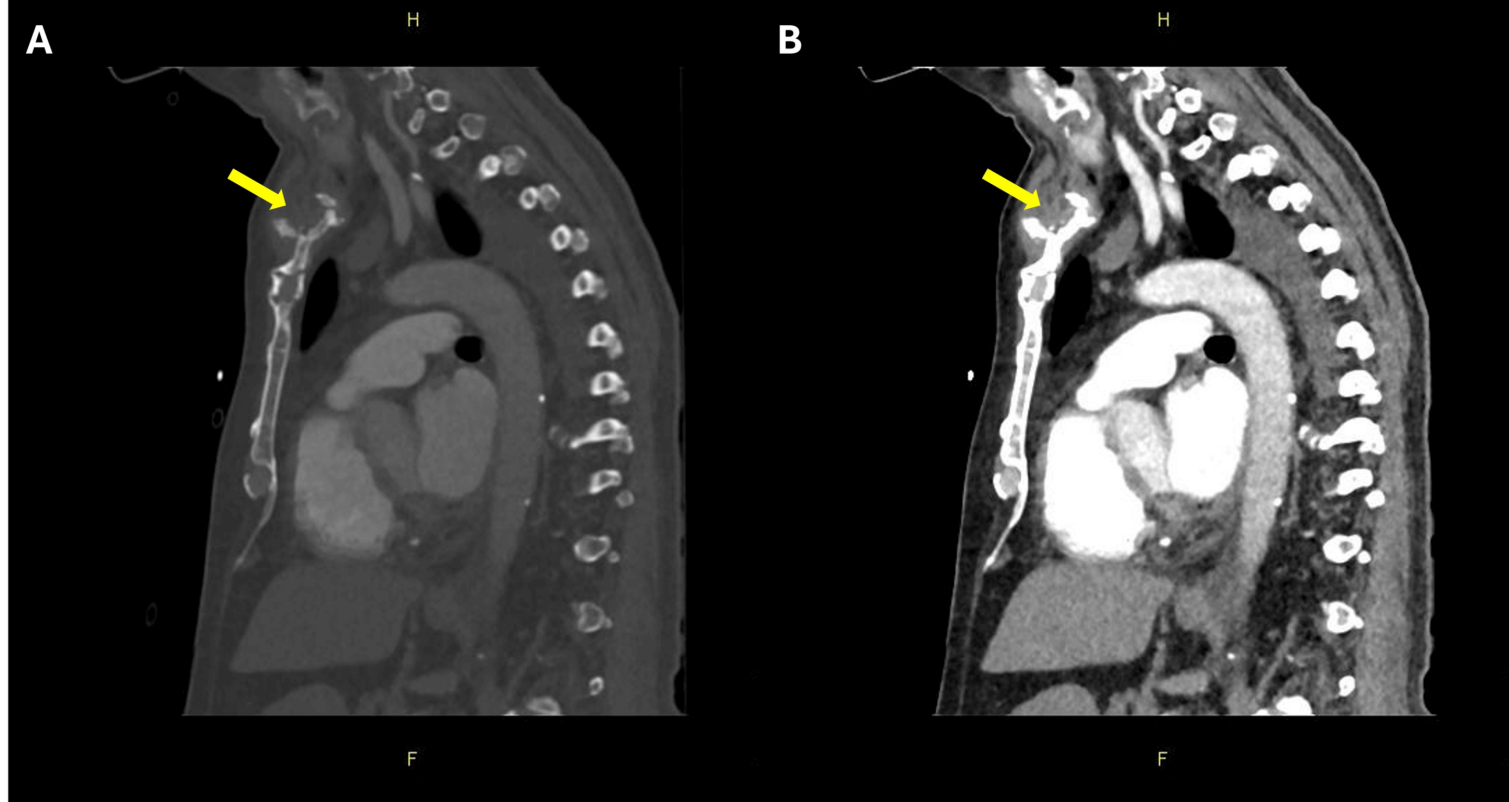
Sagittal CT images of the left sternoclavicular joint. (A) Soft tissue window demonstrating a multiloculated peri-sternoclavicular collection (yellow arrow). (B) Bone window of the same slice highlighting the cortical destruction of the medial clavicle and manubrium (yellow arrow), consistent with sternoclavicular osteomyelitis and septic arthritis
